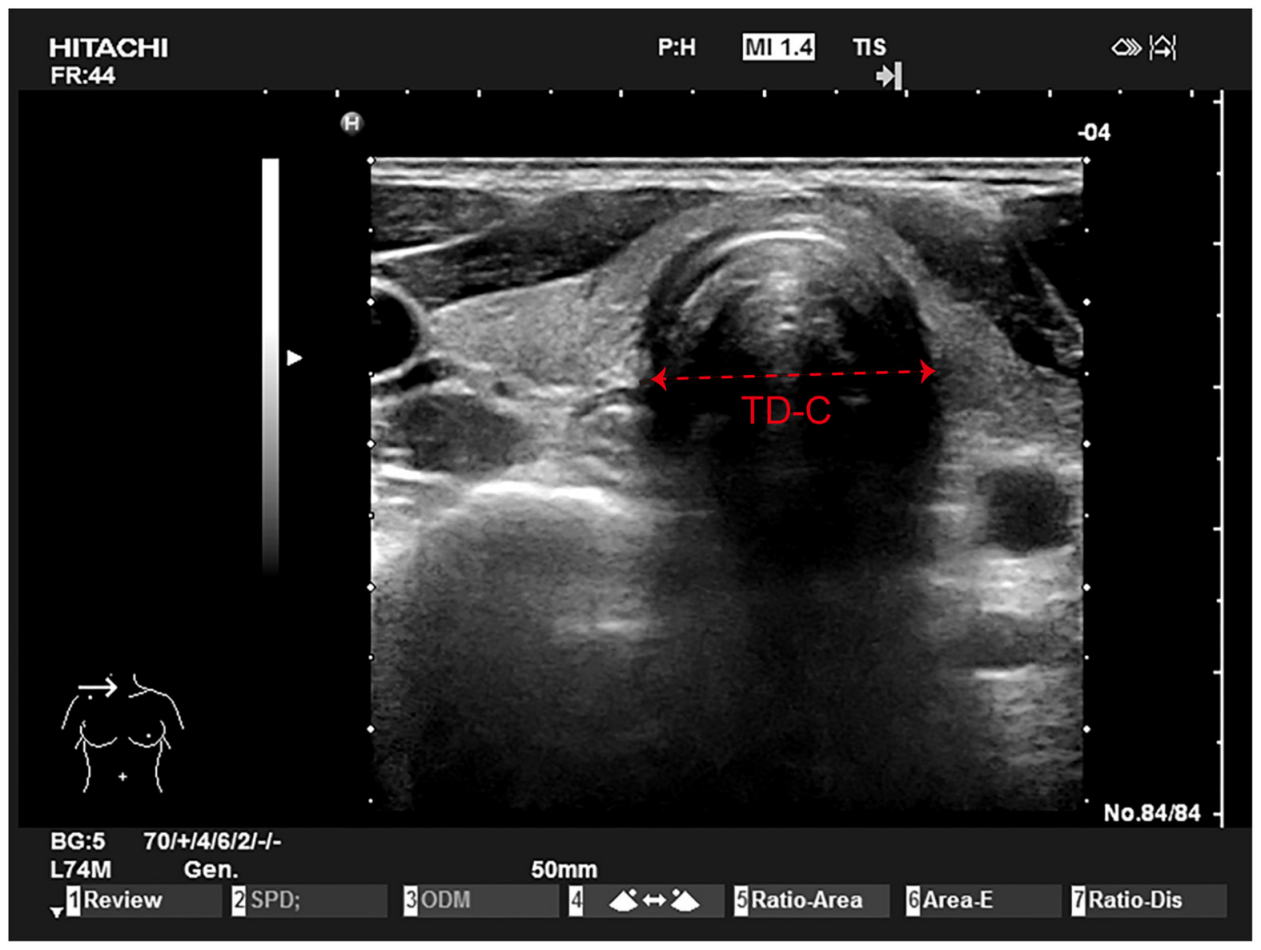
Tracheal ultrasound examination. A linear 5 to 10 MHz probe is placed perpendicularly to the neck just above the sternoclavicular junction in transverse section (left). The outer tracheal diameter is measured on this transverse view (mm).
